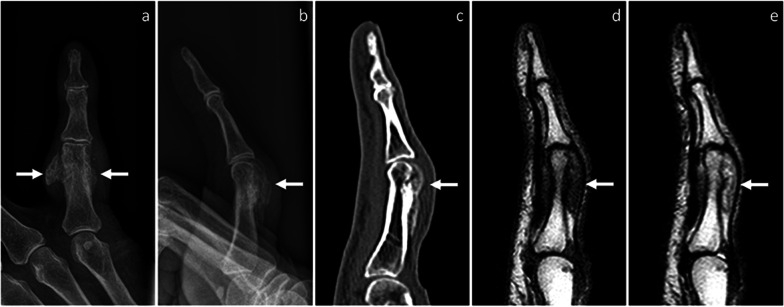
BPOP arising from the proximal phalanx of the little finger. On X-rays, frontal (a) and lateral, (b) views show a well-defined mass of heterotopic mineralization, which is contiguous to the proximal phalanx. On sagittal CT image (c), the mass is cortex-based with no cortico-medullary continuity, cortical breakthrough, or marrow extension. On MRI, the mass is hypointense on T1-weighted (d) and hyperintense on T2-weighted (e) sagittal sequences, respectively. Location and imaging findings are in keeping with BPOP. Surgical resection was performed and BPOP was pathologically proven. Arrows point at BPOP in all images
